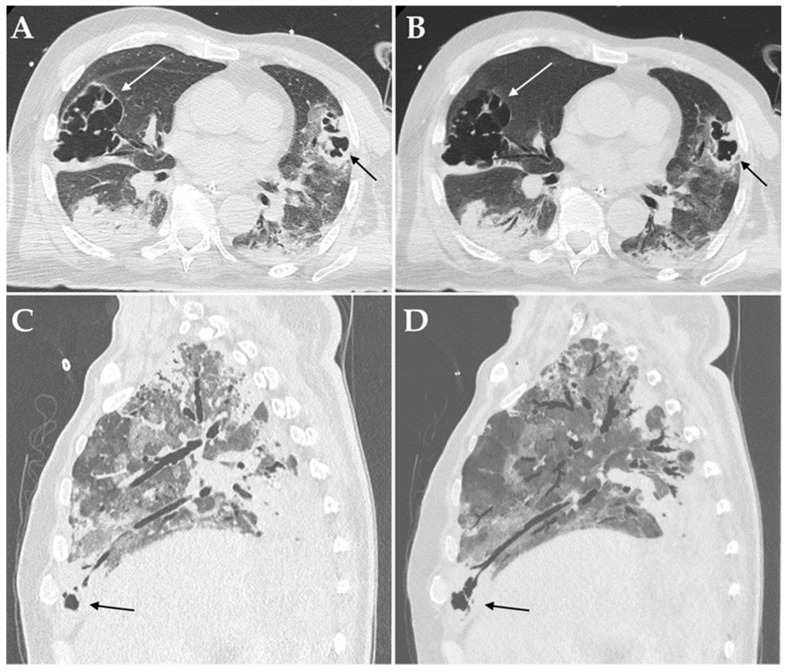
Axial HRCT images of a patient admitted to ICU with confirmed superinfection by *Aspergillus fumigatus* showing pulmonary consolidations in the lower lobes complicated by bilateral cavitations (white and black arrows in (A)); Minimum Intensity Projection (MIP) reconstruction of the same patient demonstrating communication between the cavitations and the bronchial tree (white and black arrows in (B)). Sagittal HRCT images of a patient admitted to ICU with confirmed superinfection by *Aspergillus niger* showing pulmonary consolidations in the anterior-basal segment of the right lower lobe complicated by small cavitations (black arrow in (C)); Minimum Intensity Projection (MIP) reconstruction of the same patient demonstrating communication between the cavitation and the bronchial tree (black arrows in (D)).
